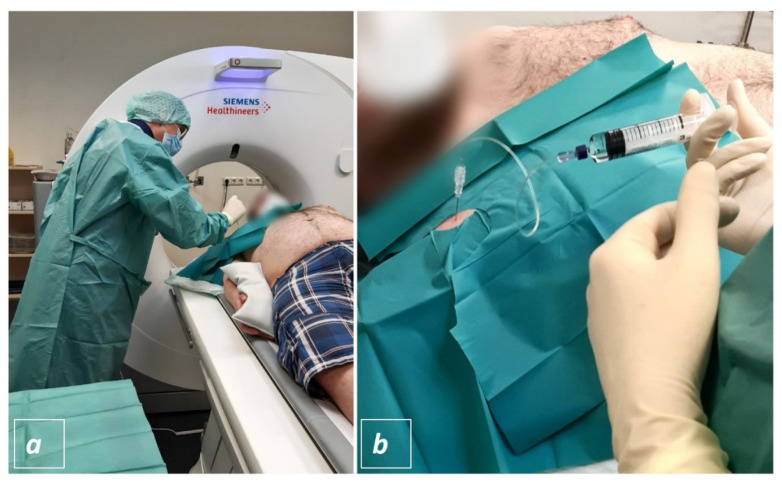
CT-guided joint injection: In shoulder injections the arm was externally rotated and this position was fixed by placing a 2 kg sandbag onto the patient’s hand. After needle pathway planning and under sterile provision the interventional radiologist advanced a spinal needle towards the joint (a). If the needle tip appeared to be intraarticular, 1–2 mL of Iopamidol were injected and the intraarticular distribution was confirmed by sequential CT. Thereafter, 10–20 mL of Gadopentetat-Dimeglumin were injected into the joint (b).
